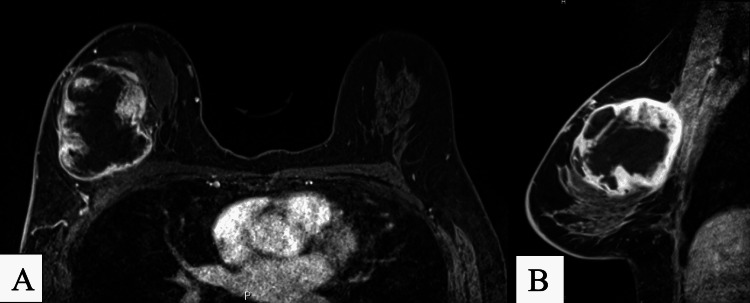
MRI performed following 25 cycles of radiation therapy (A) Axial T1 MRI demonstrating heterogenous enhancement in the mass in the central outer aspect of the right breast with central necrosis. Note the interval decrease in solid/peripheral enhancement; (B) Sagittal MRI demonstrating large mass with heterogenous enhancement interval decrease in solid component post treatment
